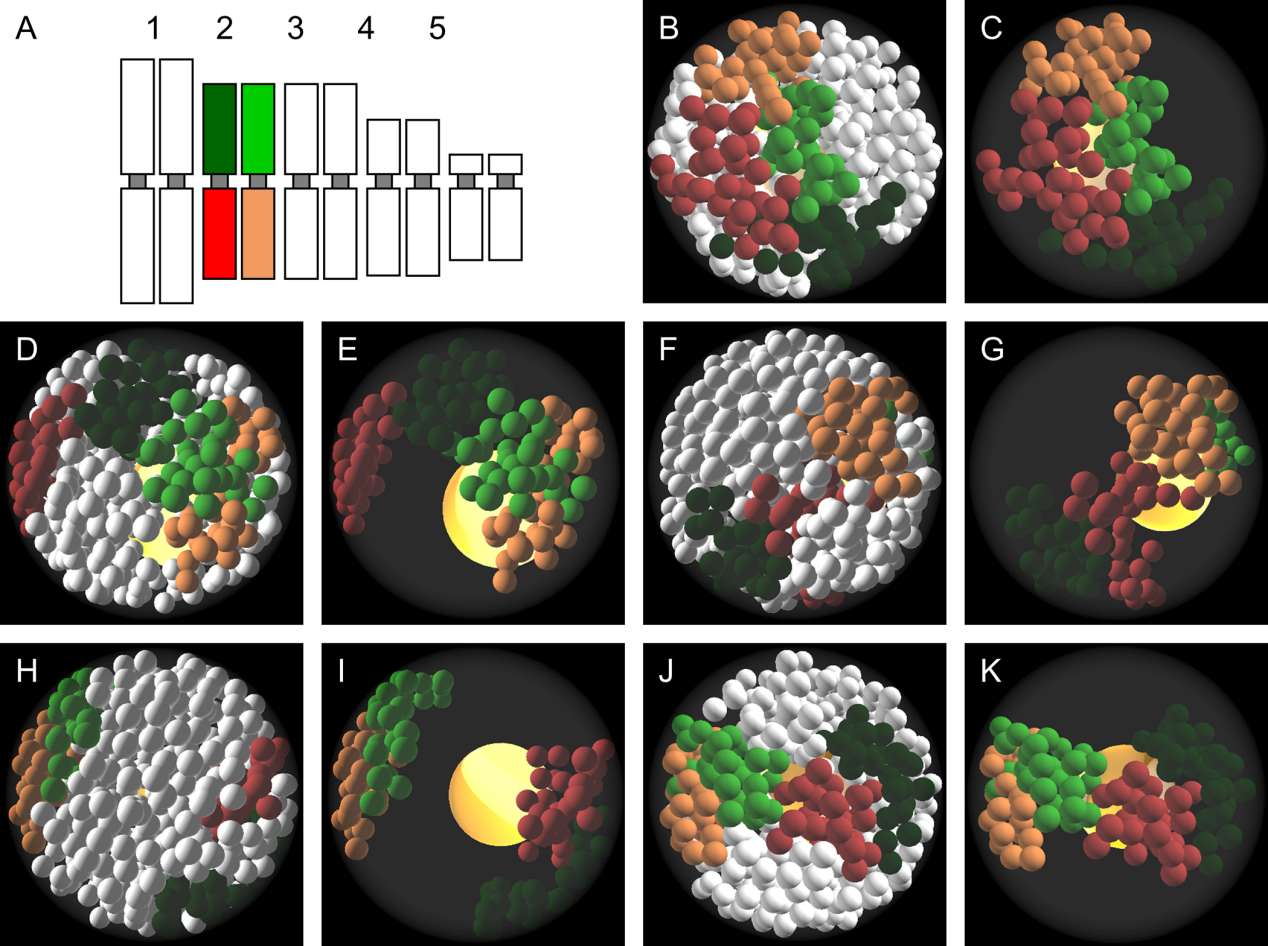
Types of homologous CT distribution patterns modelled by ChroTeMo and visualised by ChroTeVi. A–an idiogram showing the colouring of Bd2 chromosome (top arm–green, bottom arm–red). B–the nucleus with complete association of Bd2 chromosome arm territories. Chromosomes other than Bd2 are coloured white. C–the same nucleus as B. Chromosomes other than Bd2 are transparent. D–the nucleus with the association of Bd2 top arm territories. Chromosomes other than Bd2 are coloured white. E–the same nucleus as D. Chromosomes other than Bd2 are transparent. F–the nucleus with the association of Bd2 bottom arm territories. Chromosomes other than Bd2 are coloured white. G–the same nucleus as F. Chromosomes other than Bd2 are transparent. H–the nucleus with complete separation of Bd2 chromosome arm territories. Chromosomes other than Bd2 are coloured white. I–the same nucleus as G. Chromosomes other than Bd2 are transparent. J–the nucleus with association between the top arm of one Bd2 homologue and the bottom arm of another homologue. Chromosomes other than Bd2 are coloured white. K–the same nucleus as J. Chromosomes other than Bd2 are transparent. Nucleolus is visible as a yellow sphere inside the nucleus.
